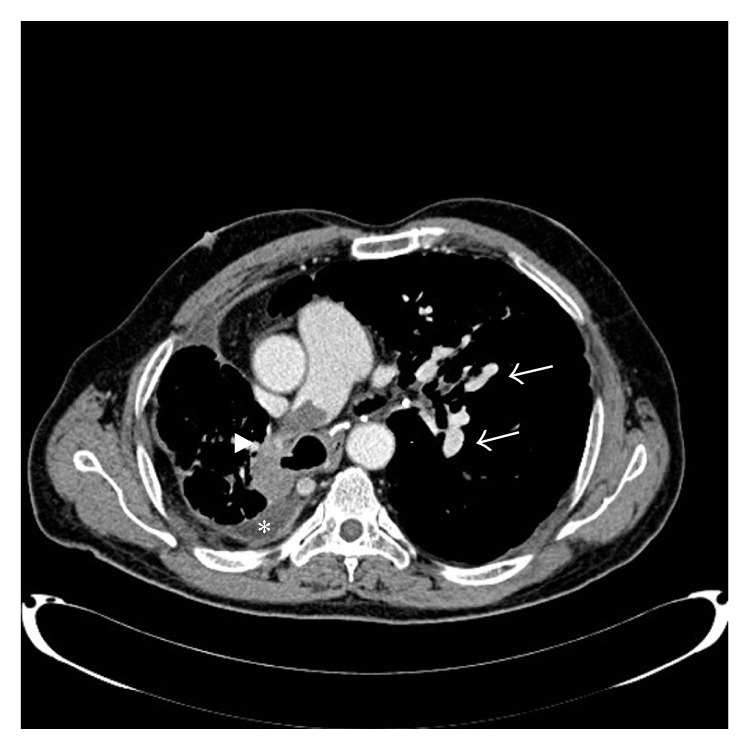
Computed tomography scan of the chest with contrast showing a pulmonary embolus in the right main pulmonary artery (arrow head), with calcified lymph nodes (arrow), in addition to right pleural effusion (asterisk).
